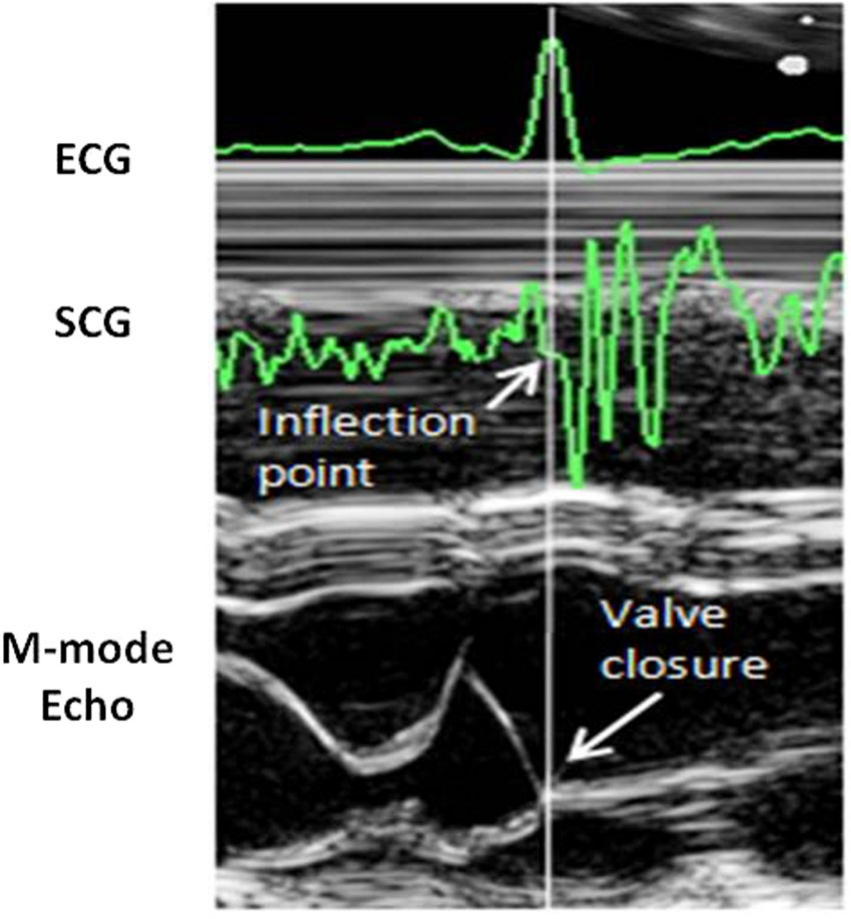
Example of SCG inflection point corresponding to the mitral valve closure. M-mode ultrasoung image from a healthy subject.
